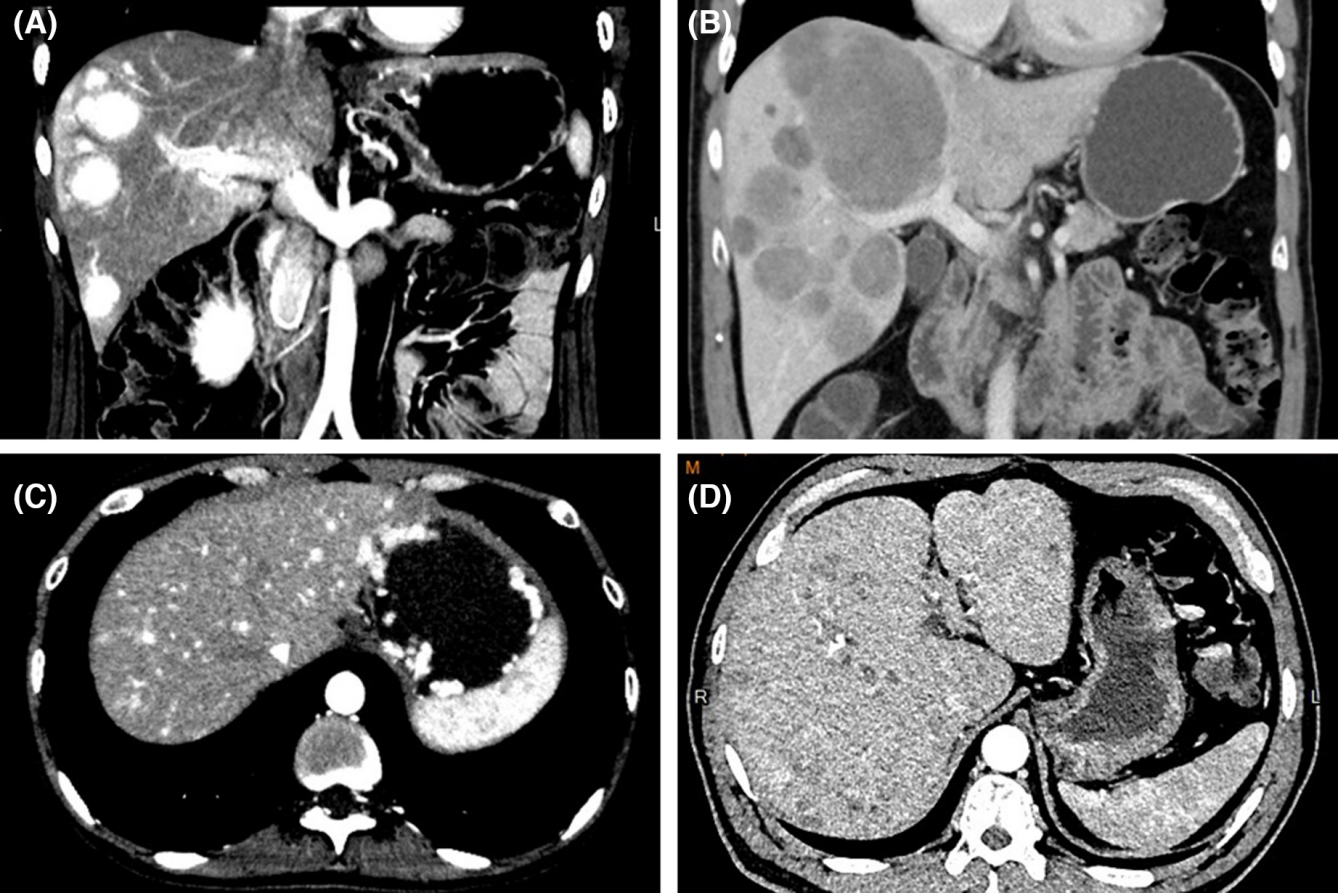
Examples of various characteristics of neuroendocrine tumor liver metastases shown in contrast CT image, including hypervascular (A, C), hypovascular (B, D), with clear tumor border (A, B) and without clear border (C, D)
